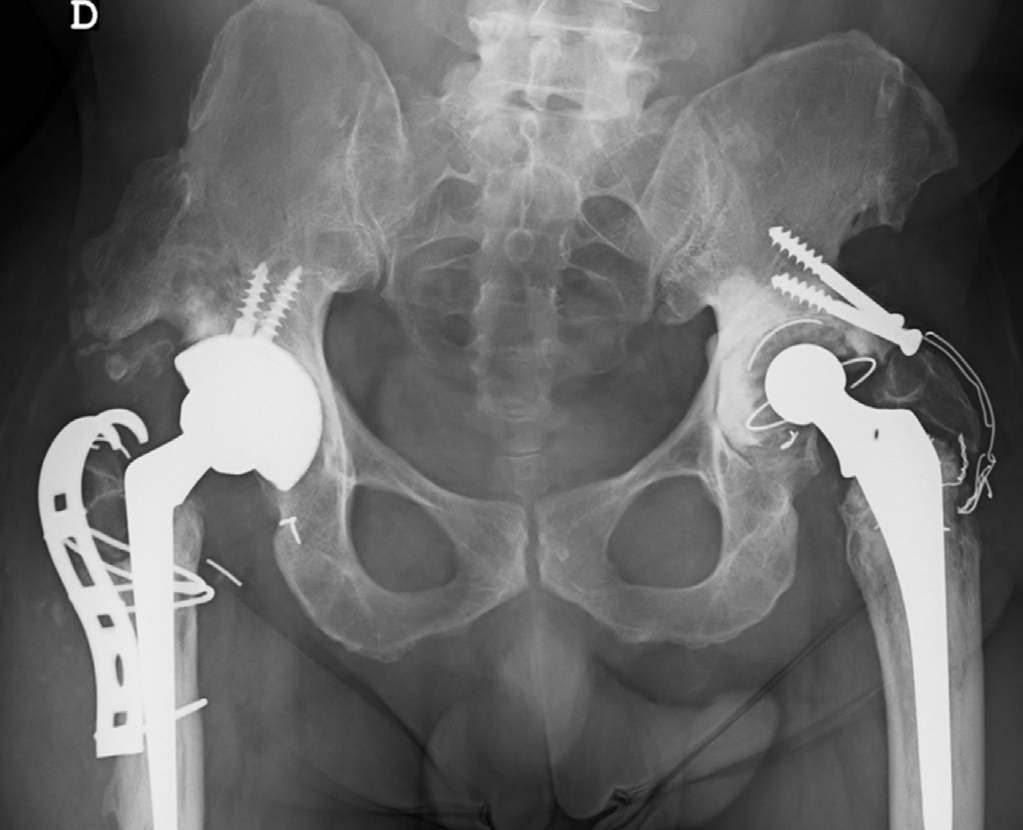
Anteroposterior radiograph of the pelvis showing the results of the revision surgery.
